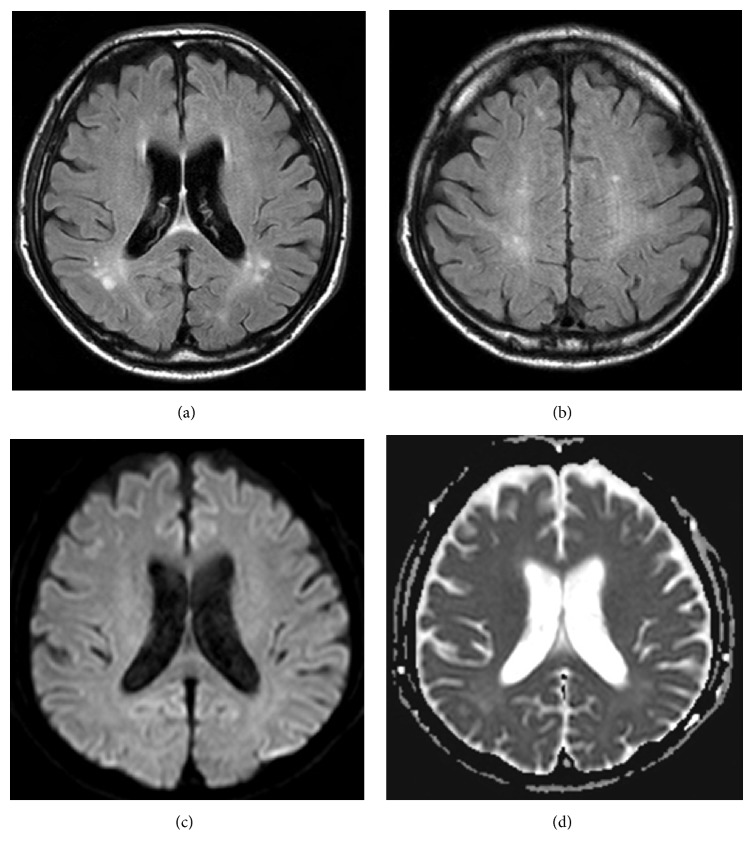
Brain MRI taken on day 29. ((a), (b)) Cranial T2-weighted FLAIR MRI showing several old small brain infarctions. (c) DWI obtained on day 29, showing no hyperintense signal alterations. (d) ADC mapping obtained on day 29, showing no increased diffusion in cerebral lesions.
